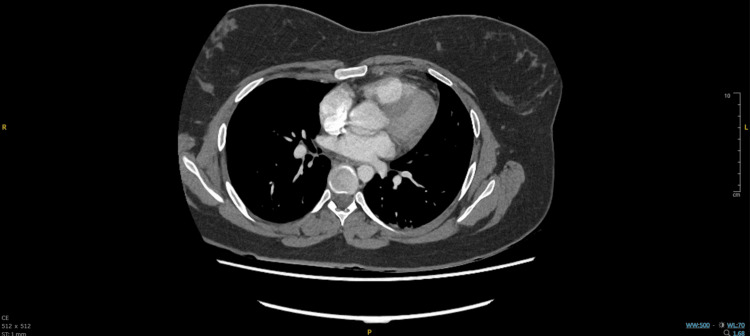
Normal contrast-enhanced CT of the pulmonary arteries (mediastinal window)
